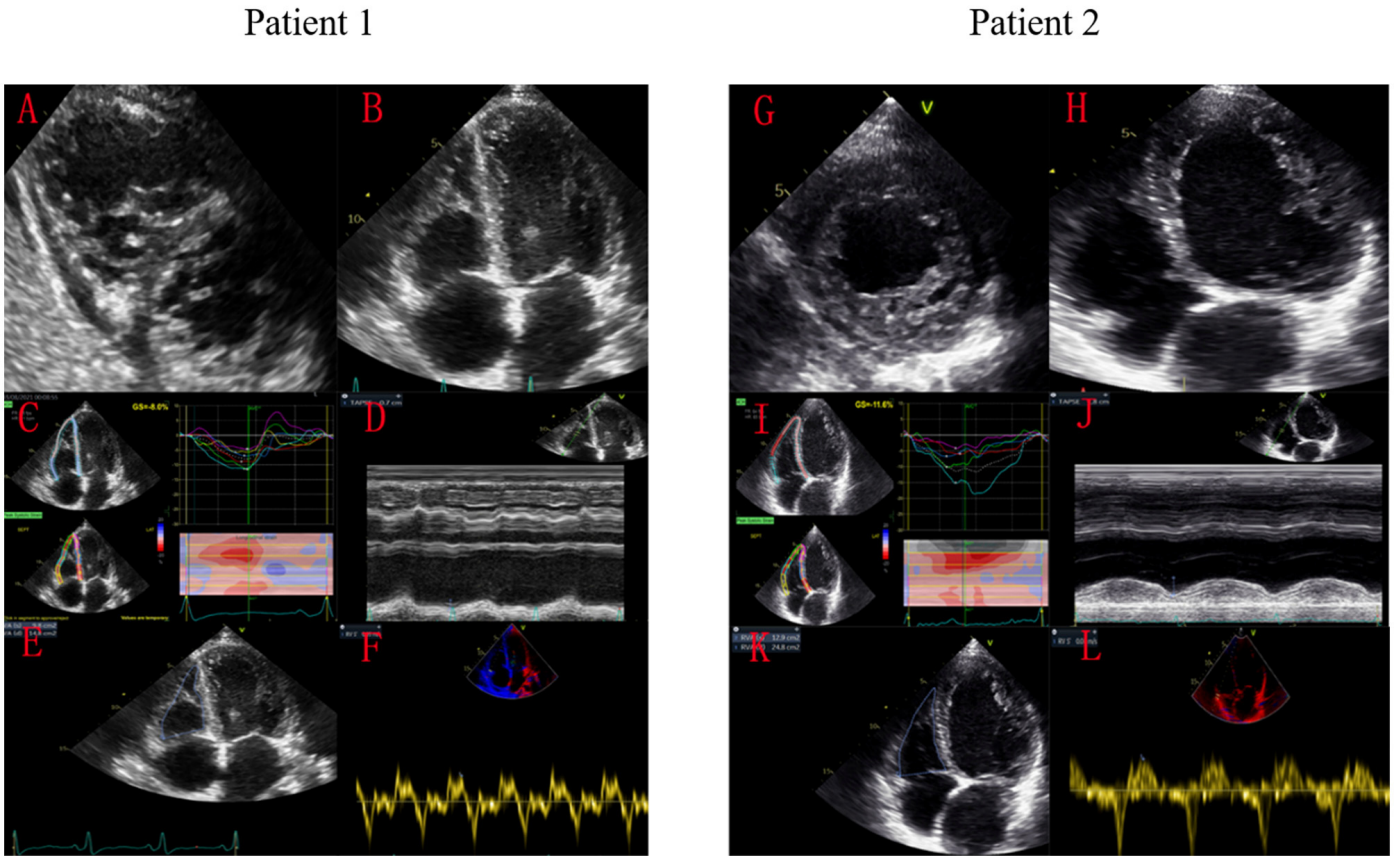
Speckle-tracking echocardiography images of two patients with LVNC and the same LVGLS. Patient 1 with significant RV dysfunction died of cardiogenic shock, while patient 2 survived for long-term after treatment. Patient 1 had significant echocardiographic manifestations of RV non-compaction (A,B). His RVGLS was −8.0% (C), TAPSE was 7mm (D), RV FAC was 24% (E), and tricuspid S′ was 0.05 m/s (F). Patient 2 only had LV echocardiography manifestations of ventricular non-compaction (G,H). His RVGLS was −11.6% (I), TAPSE was 18 mm (J), RV FAC was 47% (K), and tricuspid S′ was 0.07 m/s (L).
